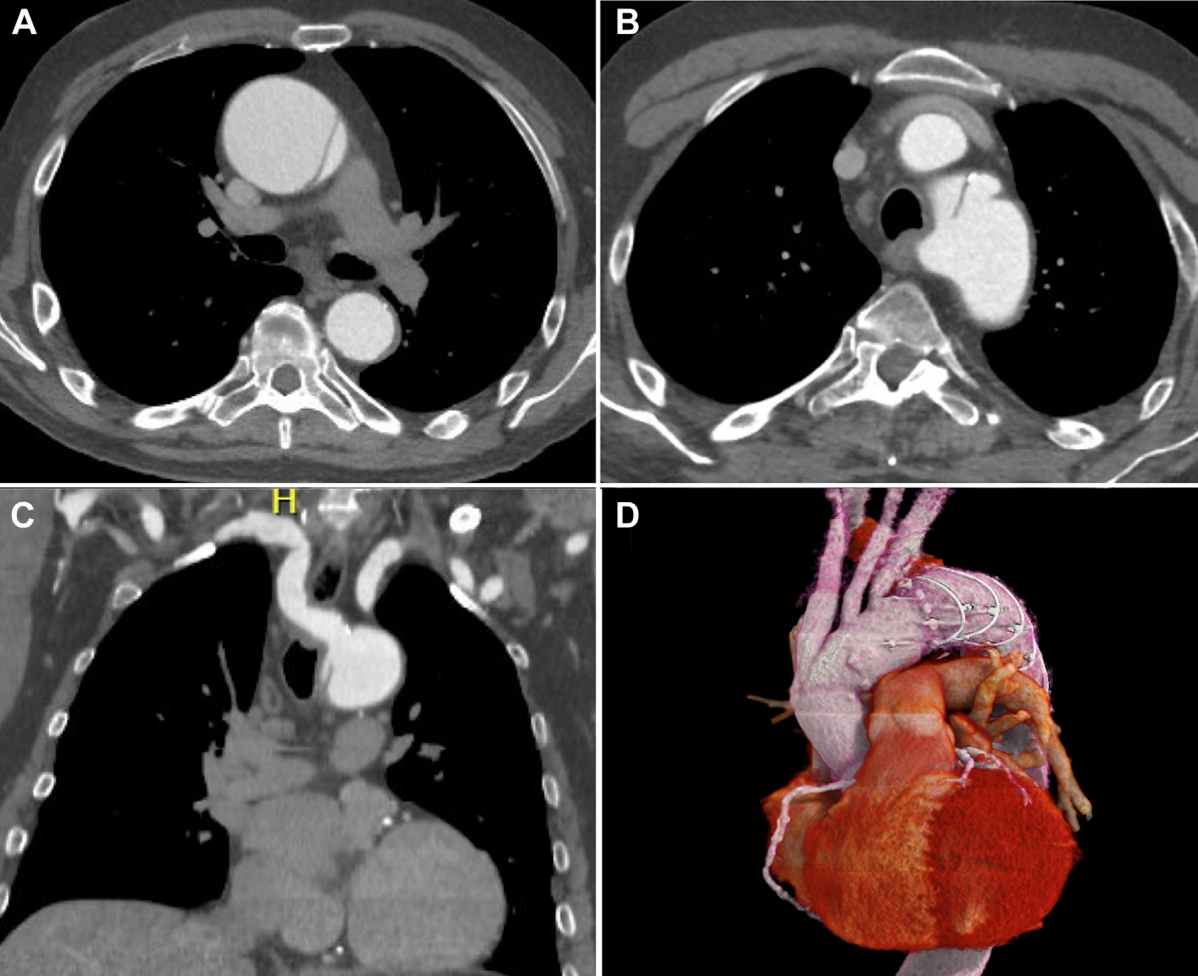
(A) Preoperative computed tomography (CT) scan showing an acute type A aortic dissection. (B) Preoperative CT scan showing the entry tear originating at the level of the Kommerell diverticulum. (C) Preoperative coronal CT reconstruction showing the origin and course of the aberrant right subclavian artery. (D) Postoperative 3-dimensional CT reconstruction showing the hybrid frozen elephant trunk and all its branches and the thrombosed Kommerell diverticulum.
